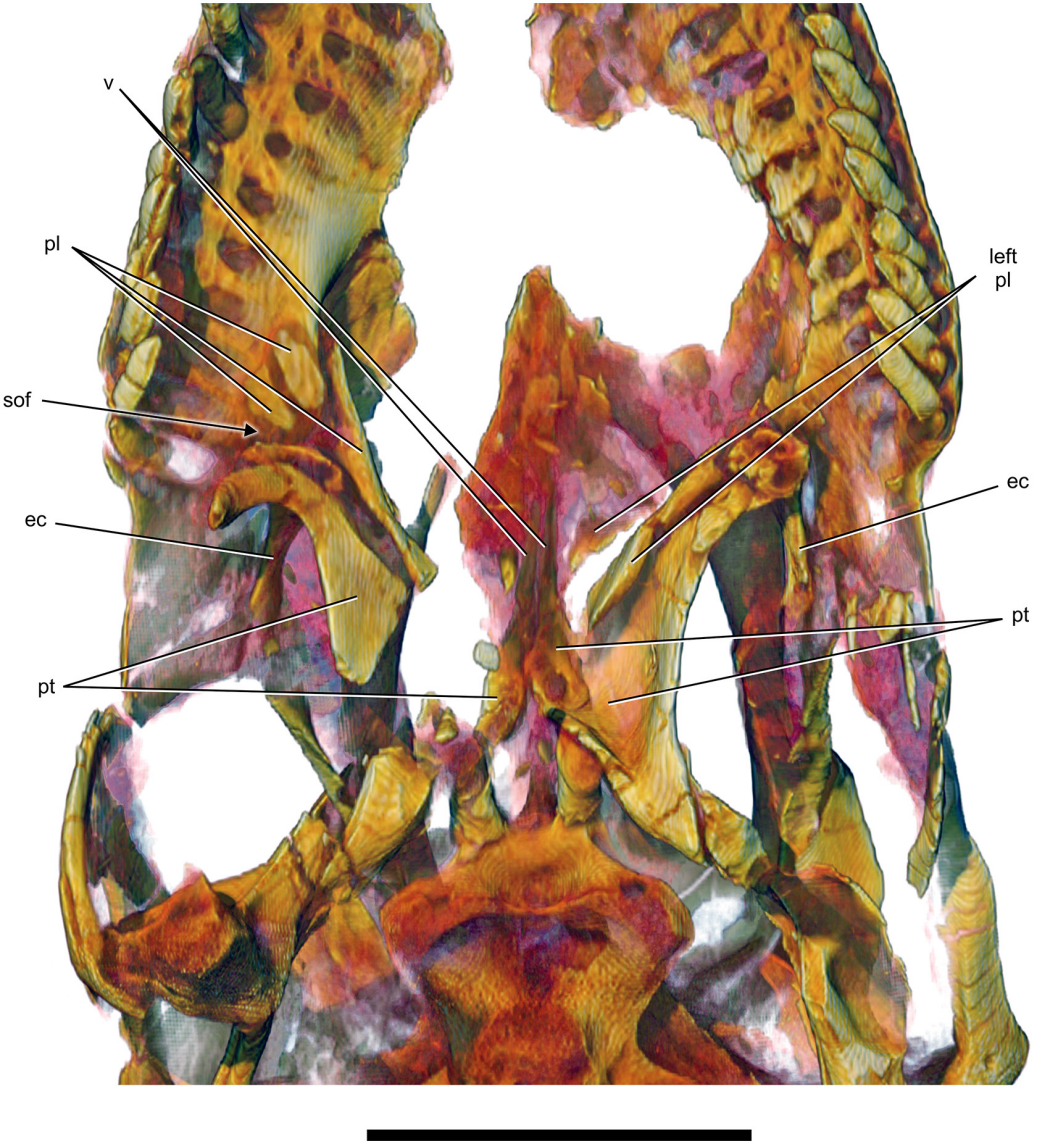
Palate of *Sarmientosaurus musacchioi* gen. et sp. nov. (MDT-PV 2). Computed tomography-based digital visualization in ventral view indicating palatal bones (ectopterygoids, palatines, pterygoids, and vomers) and the right suborbital fenestra. Abbreviations see text. Scale bar = 10 cm.
